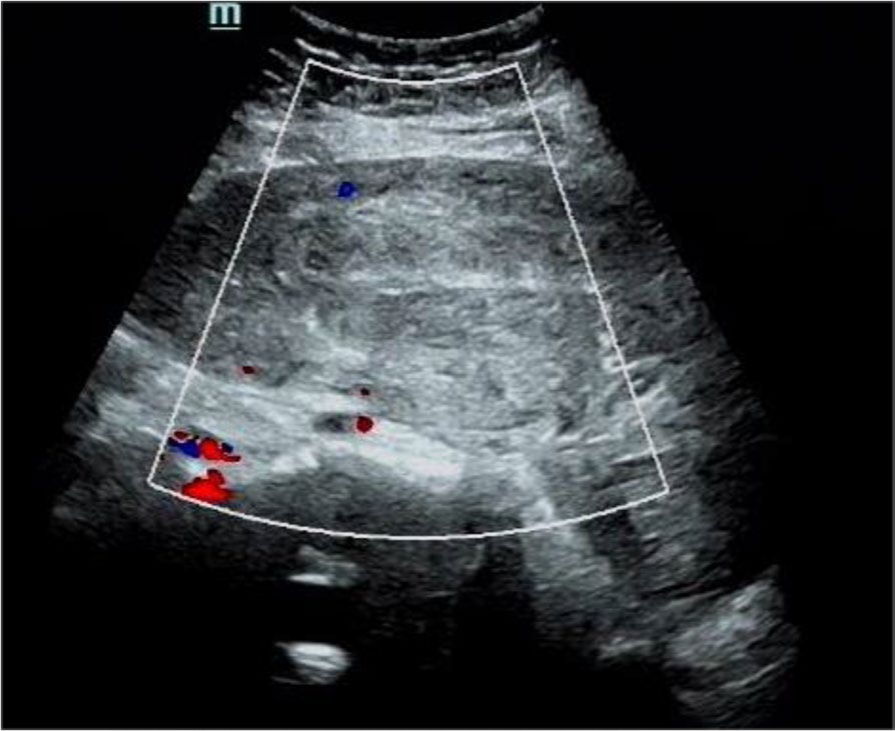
Postoperative ultrasonography showed that the incision of the posterior wall of the uterus had recovered well, the myometrium was continuous, the intrauterine line was clear, and there was no hemorrhage or placental membrane residue in the uterine cavity
